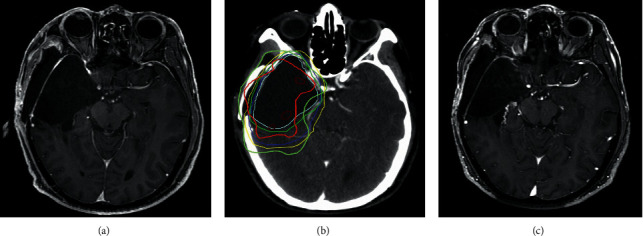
Example of patient without NERF. A 63-year-old male with pathologically confirmed anaplastic astrocytoma in the right temporal lobe. His OS was 790 days. Axial contrast-enhanced T1-weighted image 72 h after resection (a). Axial contrast-enhanced CT image with treatment-planning overlay shows the volumes treated with 60 Gy (red outline) and 50 Gy (yellow outline) (b). There was no new developed enhancing lesion within and outside radiation field in follow-up MRI 6 months after completion of radiotherapy (c). NERF, new enhancement beyond the radiation field.
